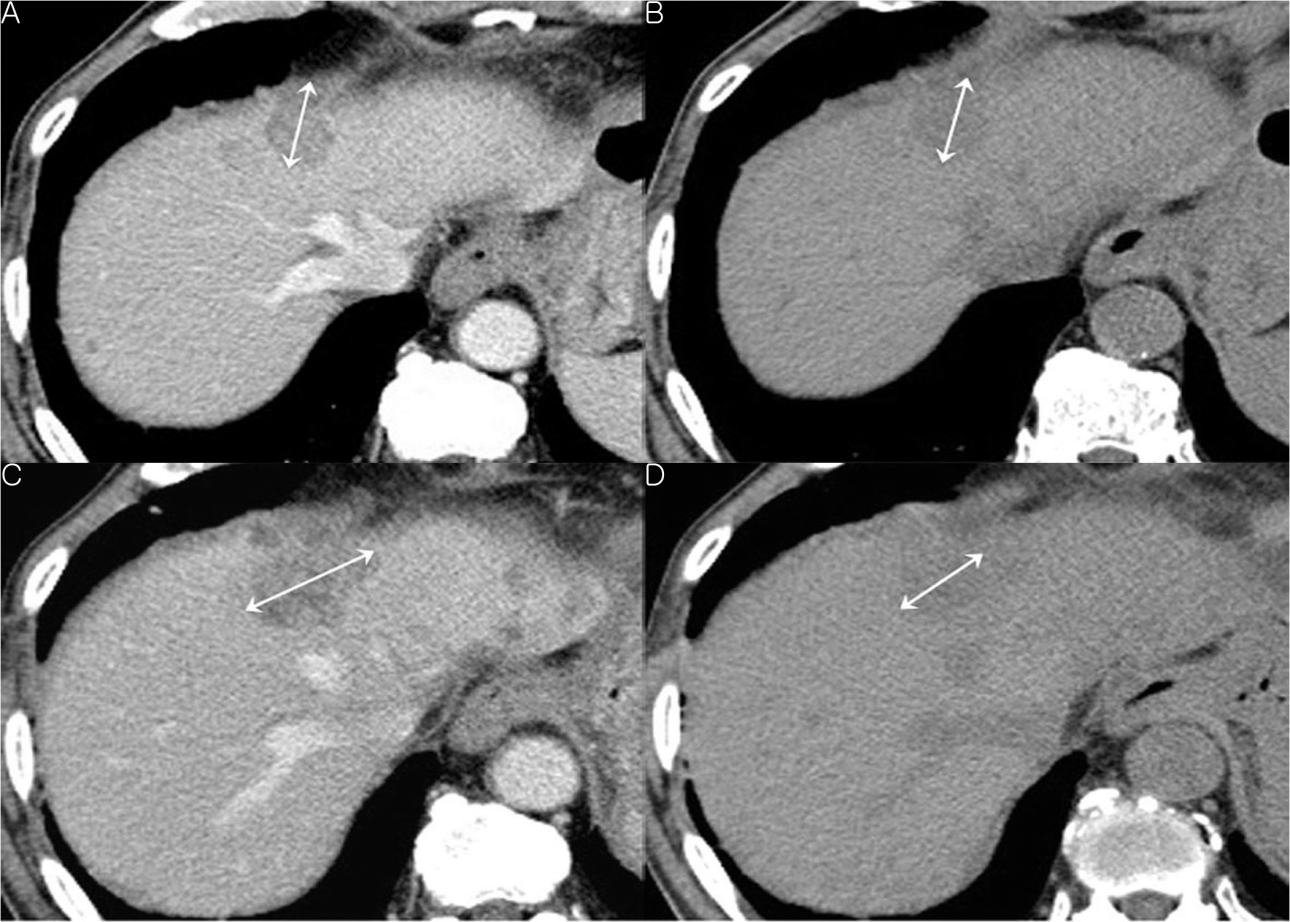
Seventy-two-year-old man who had known liver metastasis from gastric cancer. a. On the portal venous phase, hepatic metastasis is seen in segment 4 of the liver. b. The size of the lesion appears similar in the non-enhanced CT image. This lesion was measured as 30 mm both in the contrast-enhanced CT (a) and non-enhanced CT (b) images. (c, d) On follow-up CT after 3 months, the lesion had increased in size. It was measured as 47 mm (57% interval increase) on contrast-enhanced CT, which suggested progression of disease (c). However, on non-enhanced CT, the mass was measured as 32 mm (7% interval increase), which suggested stable disease.
